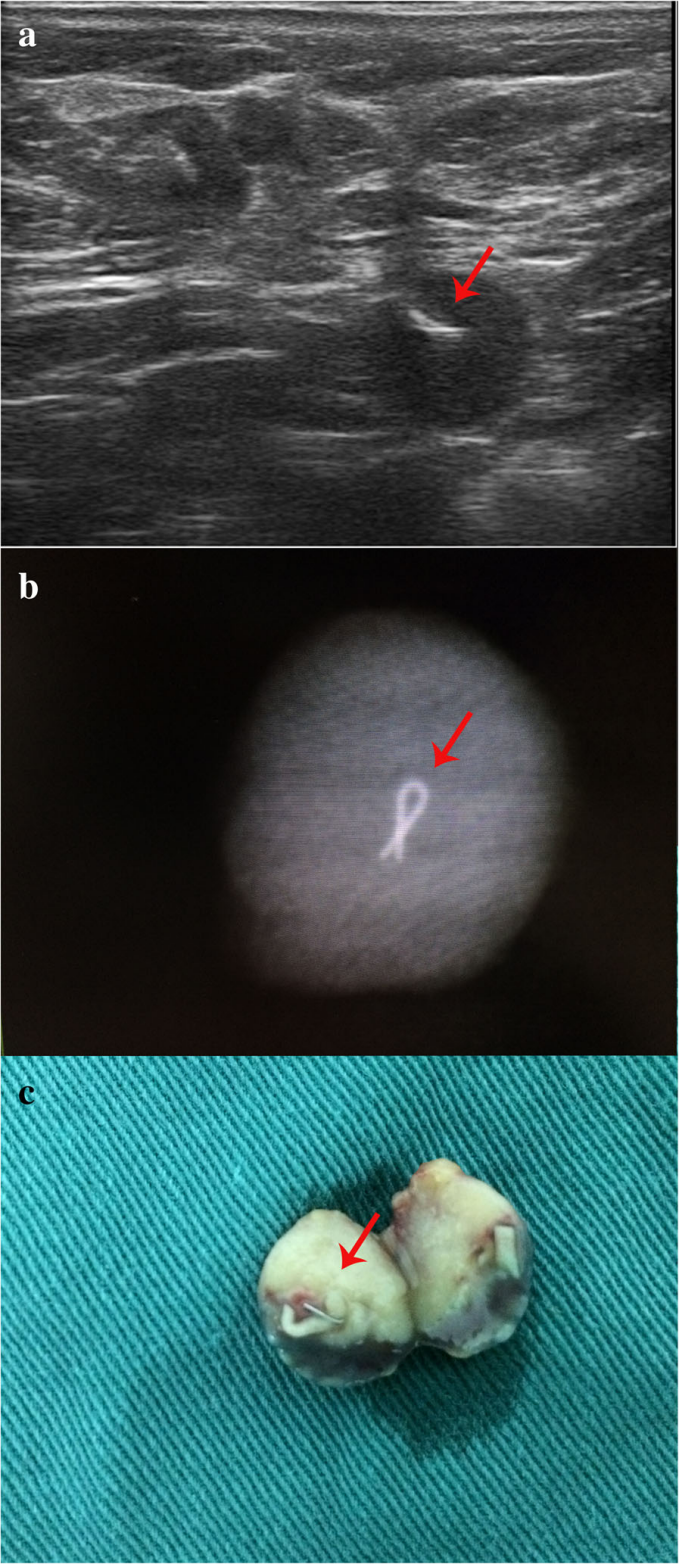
Gray-scale ultrasound examination, X-ray examination, and dissection used to confirm the insertion of a titanium clip into the SLN. a The titanium clip (*arrow*) was shown with high-echo on gray-scale ultrasound. b The titanium clip (*arrow*) was shown with high density on X-ray imaging. c The titanium clip (*arrow*) can be viewed macroscopically after dissecting the dyed SLN
